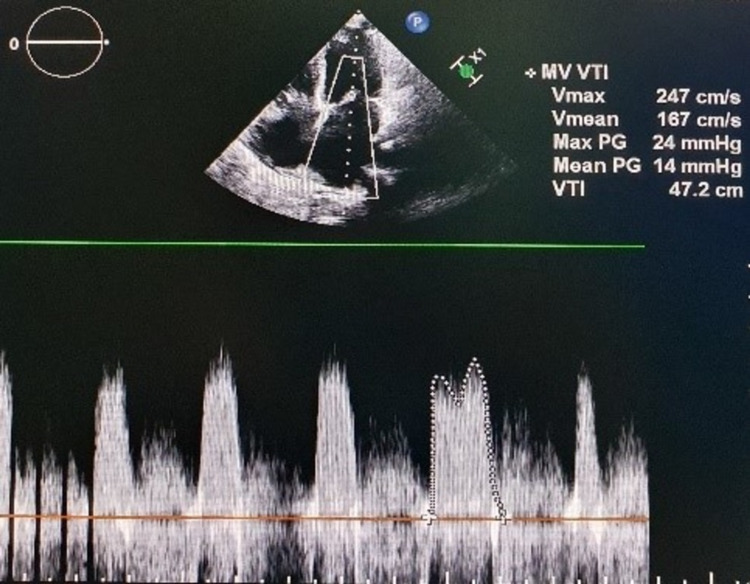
Apical four-chamber view showing a mean pressure gradient of 14 mmHg across mitral valve depicting severe mitral stenosis.
